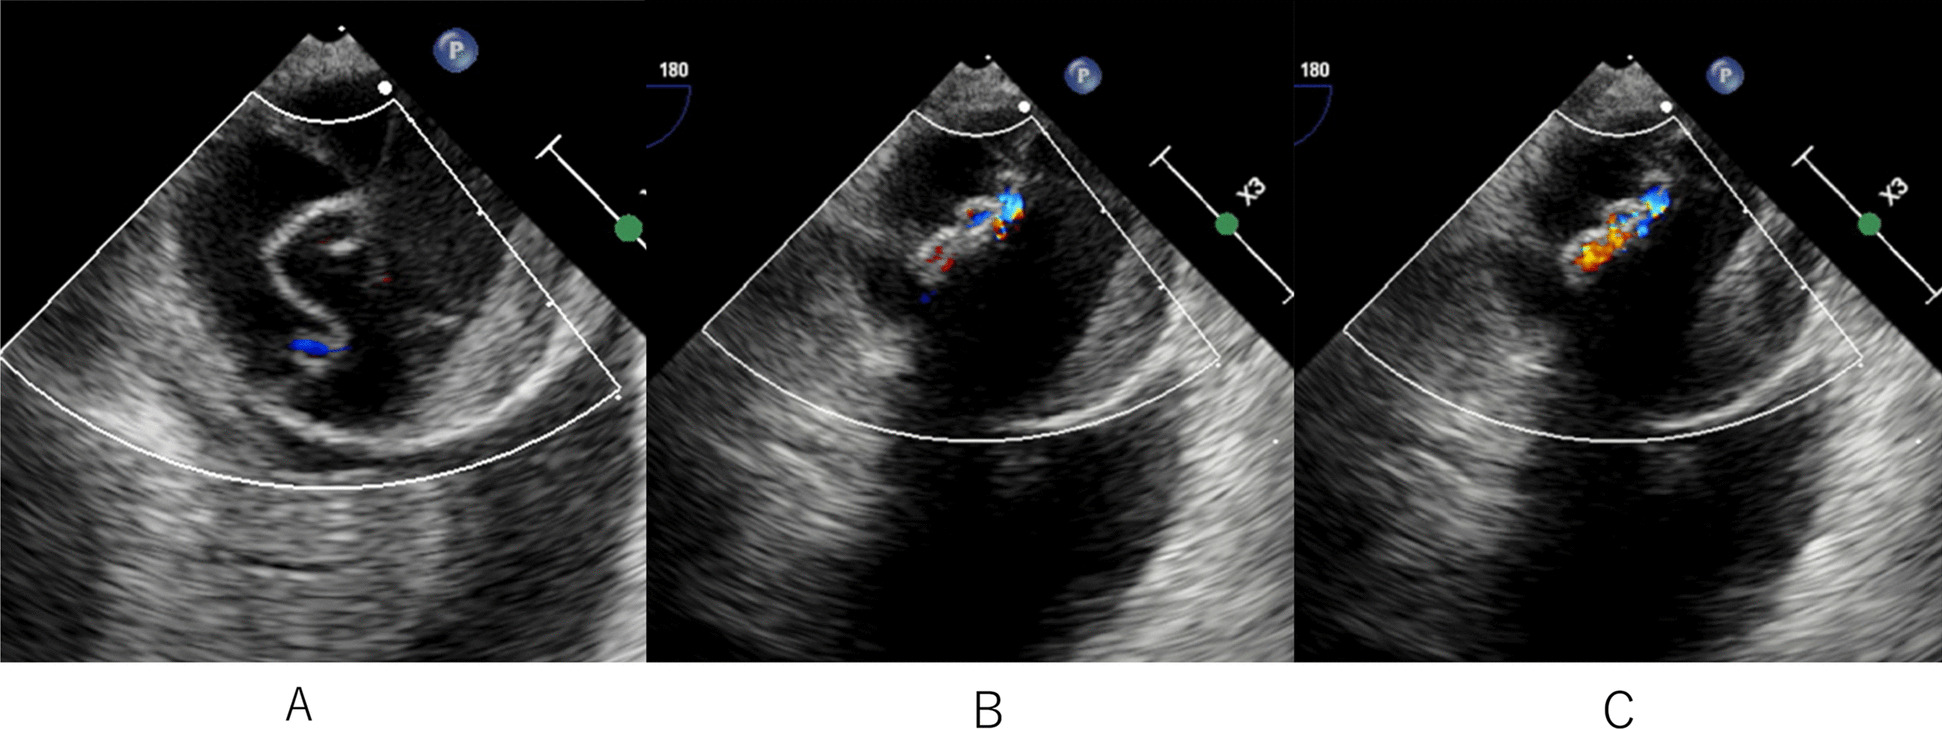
Transesophageal echocardiography (TEE) conducted on postoperative day 2. A The true lumen of the aorta was sufficiently dilated and did not compress the partial ET. B TEE revealed the stenosis of the distal skirt of Partial ET with 5.0 L/min of ECMO flow. C The stenosis was relieved when the ECMO flow was lowered to 2.0 L/min
